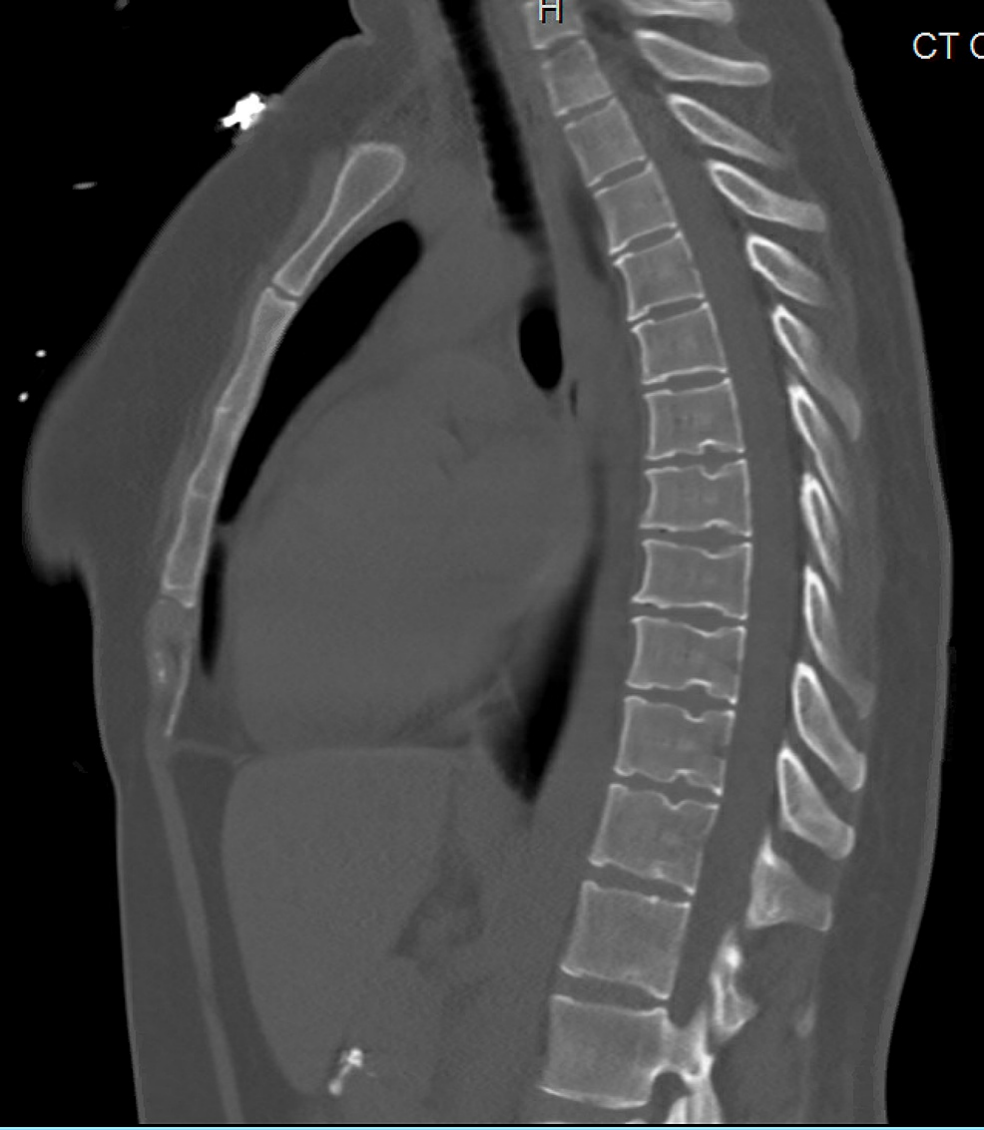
CT of the thoracic spine is not suggestive of osteolytic lesions
